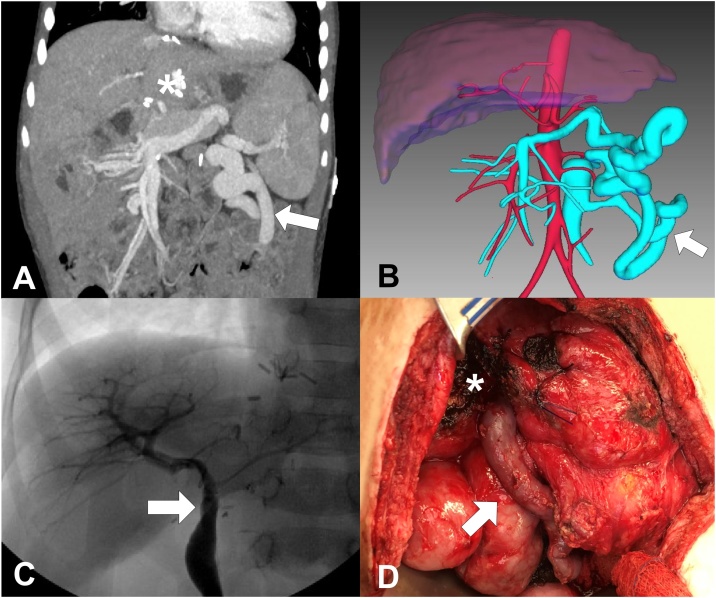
A) CT scan before the revision of the MRB showing a large splenorenal collateral vein (arrow), and the Rex recess (*). B) 3D reconstruction before the revision of the MRB showing a large splenorenal collateral vein (arrow). C) Intraoperative portogram performed during the revision of the MRB (arrow), showing a normal intrahepatic portal system. D) Image showing the re-do MRB (arrow) that was performed using a splenorenal collateral vein as an autologous venous conduit, Rex recess (*).
